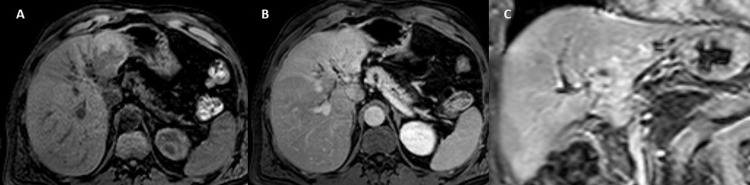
MRI shows heterogeneous hyperenhancement after extracellular contrast ({A} axial T1w pre, {B} axial T1w post-Gd portal phase, and {C} coronal T1w post-Gd delayed phase). T1w post-Gd: T1-weighted post-gadolinium
